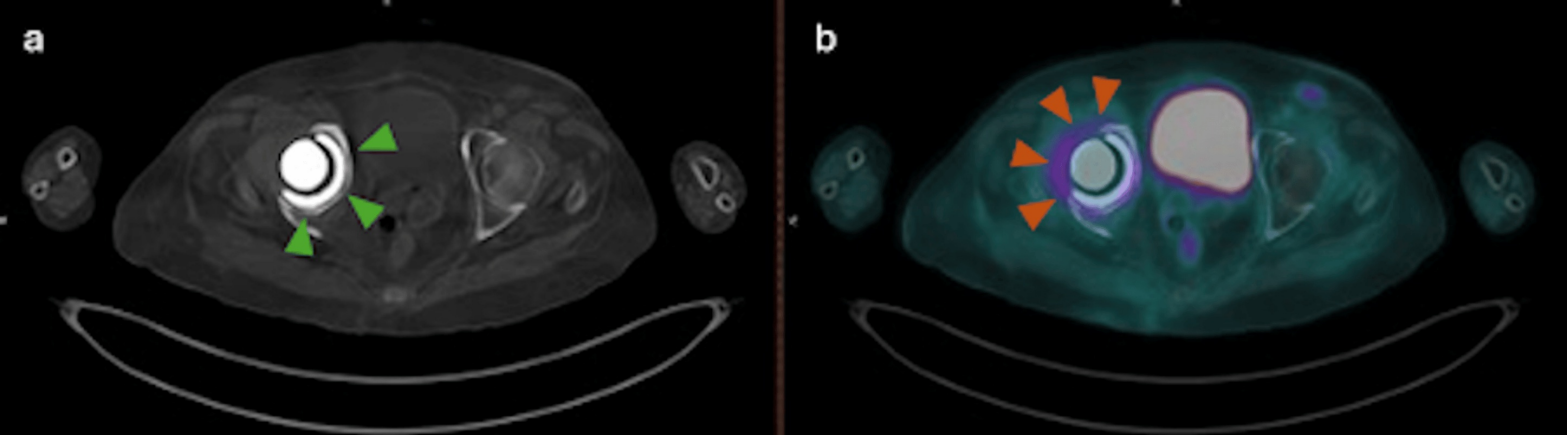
(a) Axial CT of the pelvis showing the right hip prosthesis (green arrowheads). (b) Axial 18F-FDG PET showing increased uptake around the prosthesis (orange arrowheads), consistent with inflammatory rather than infectious activity.
